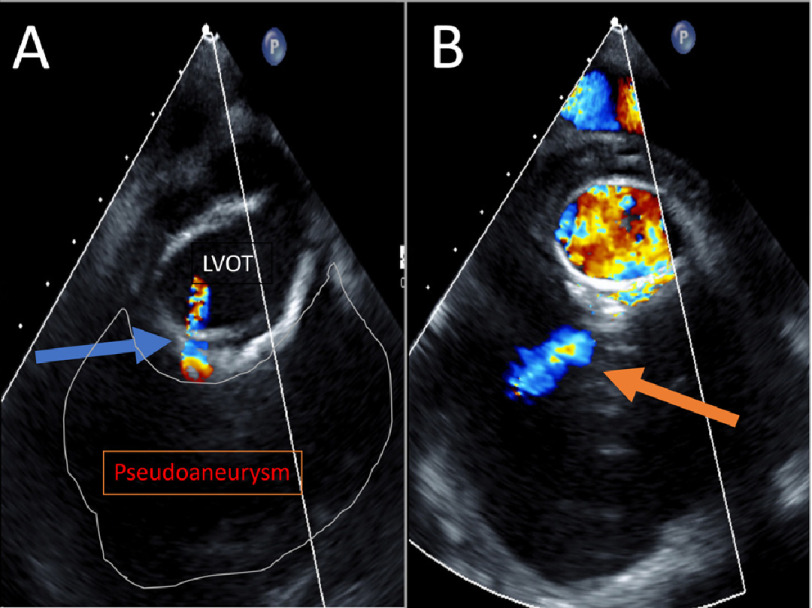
(A) TEE image with color flow doppler reveals flow between LVOT and pseudoaneurysm through a fistulous connection. (B) TEE image showing color flow doppler within pseudoaneurysm.
